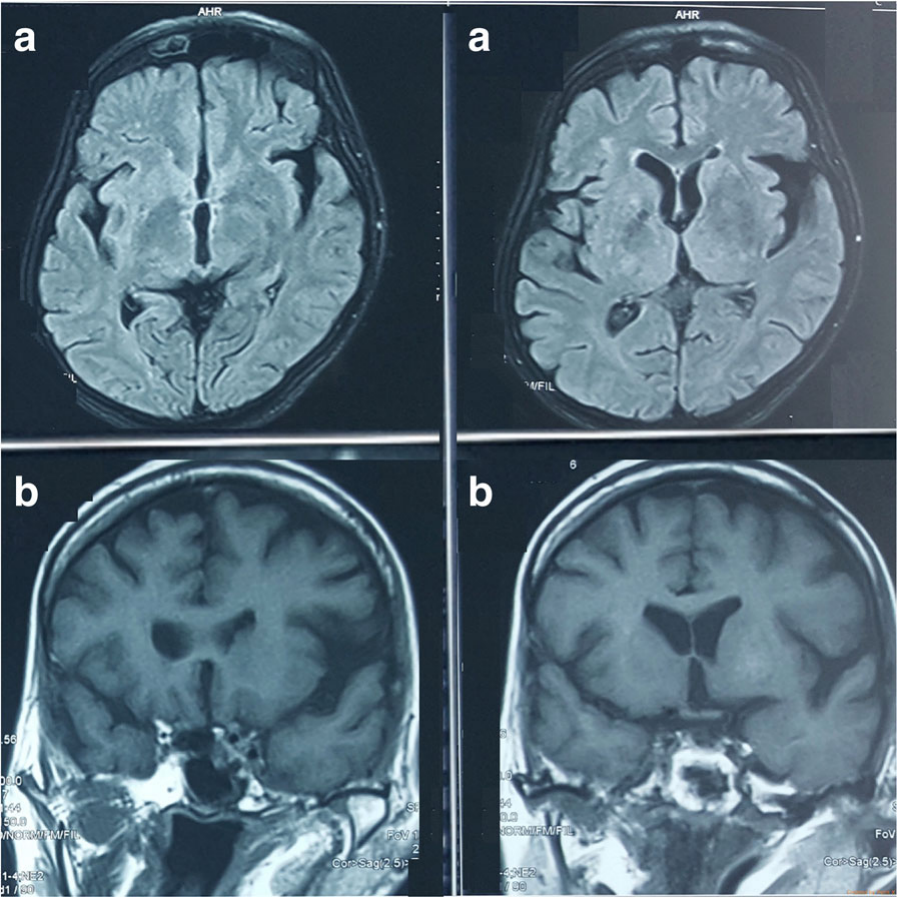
a: MRI on day 3 showing slightly low signal intensity of left side basal ganglia on T2- weighted images. b: MRI on day 3 showing slightly high signal intensity of left side basal ganglia on T1- weighted images
